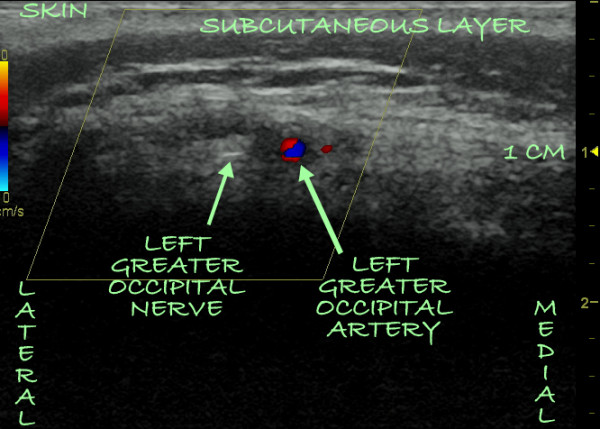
A Doppler ultrasound image of the left occipital nerve and artery as they pierce the substance of the trapezius muscle side-by-side 1 cm from the skin.
